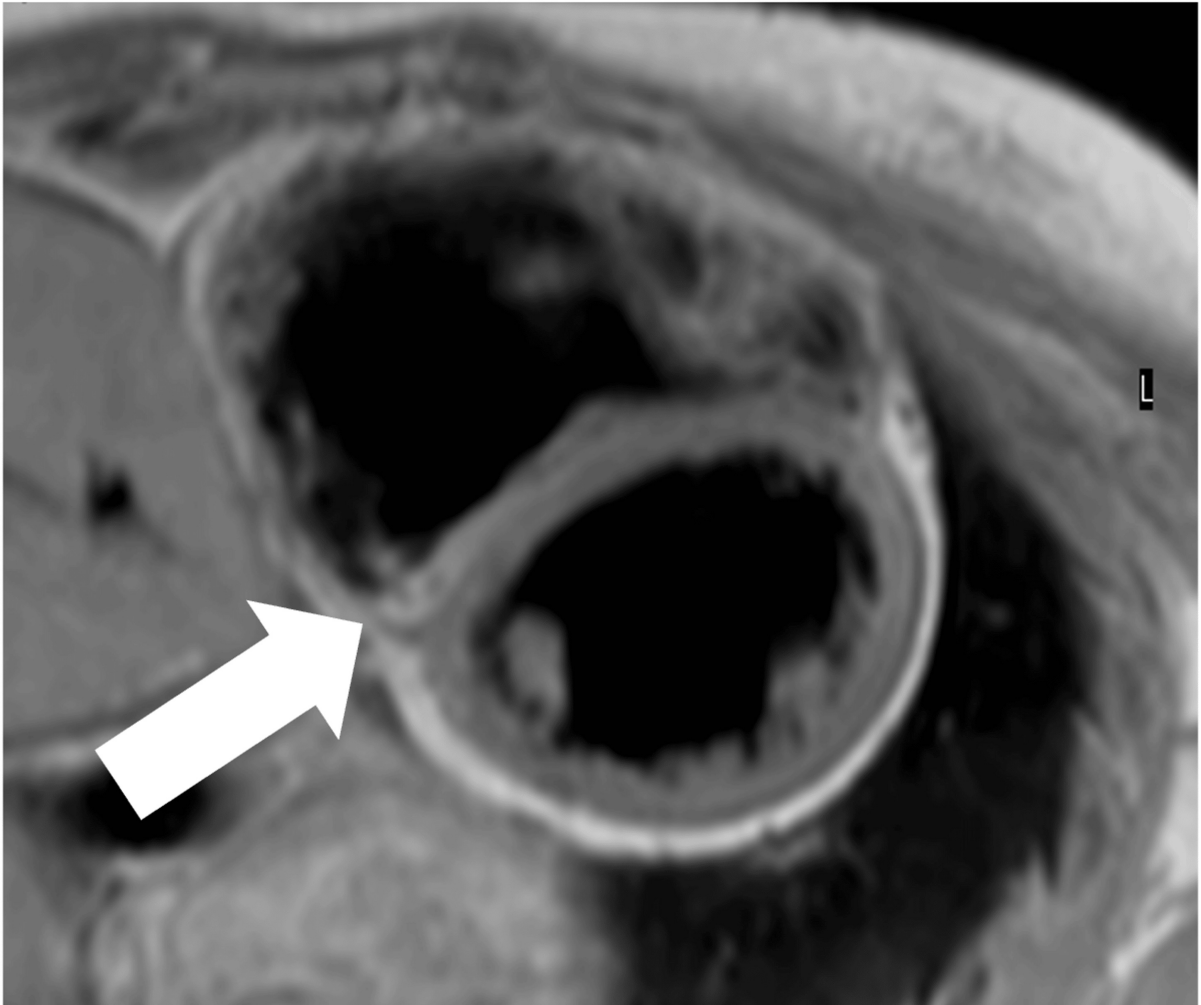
Cardiac magnetic resonance imaging of case 1 at diagnosis T1-weighted magnetic resonance imaging showing fatty replacement of the myocardium of the right ventricle (bold arrow)
